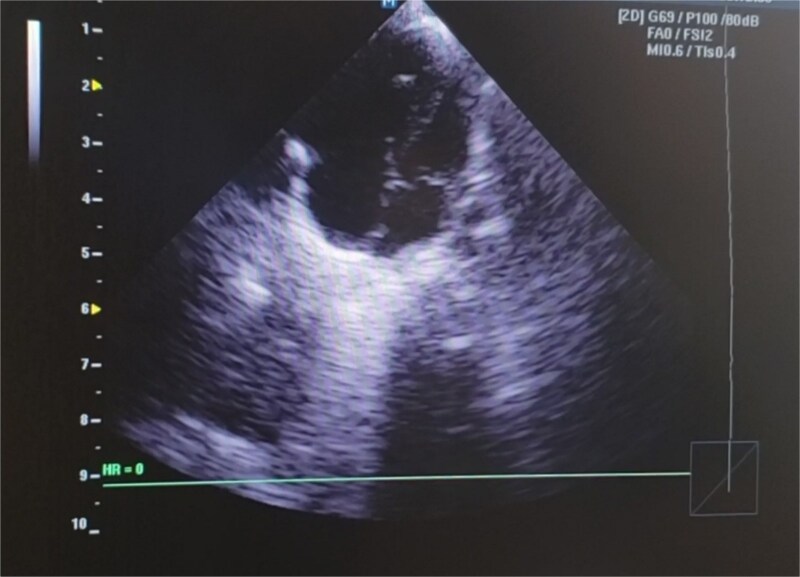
Dilated right atrium and ventricle are visible in the echocardiographic image of the index baby, which is mainly due to severe PPHN.
